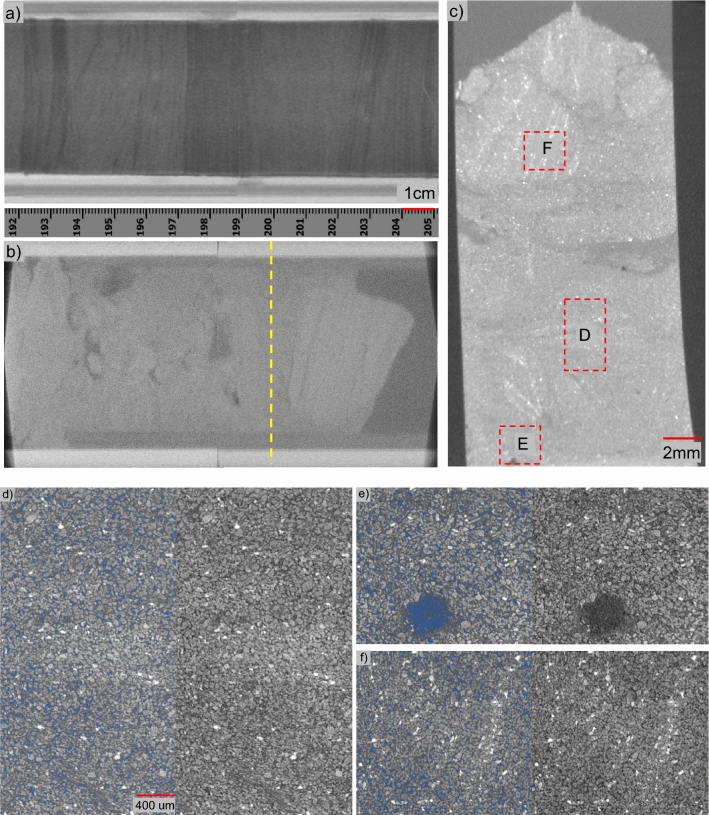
CT scan images of pressure cores at different times and scales. Figure (a) is a sectional view of a portion of core H005 5FB-3 scanned on board soon after the drilling of the pressure core, May 2017^29^; figure (b) is a sectional view of the same pressure core scanned with the industrial CT scanner (the remaining segments of the 30-cm long pressure core after three cuts), Dec 2019; figure (c) is an overview of the mini-core (Sample #3, Feb 03, 2020) drilled from the left-hand portion of the core shown in (b); figure (d) is high-resolution image of the portion of Sample #3 (noted as D on c: Jan 31-Feb 03, 2020); figure (e) is high-resolution image of the portion of Sample #3 (noted as E on c: Feb 03–05, 2020); and figure (f) is high-resolution image of the portion of Sample #3 (noted as F on c: Feb 05–07, 2020). Scales in (a) and (b) are provided by the ruler; (d), (e), and (f) share the same scale bar; (d), (e), and (f) show raw CT images on the right and the same image with hydrate false-colored in blue. Note that this false color was assigned purely according to the pixel grayscale values.
